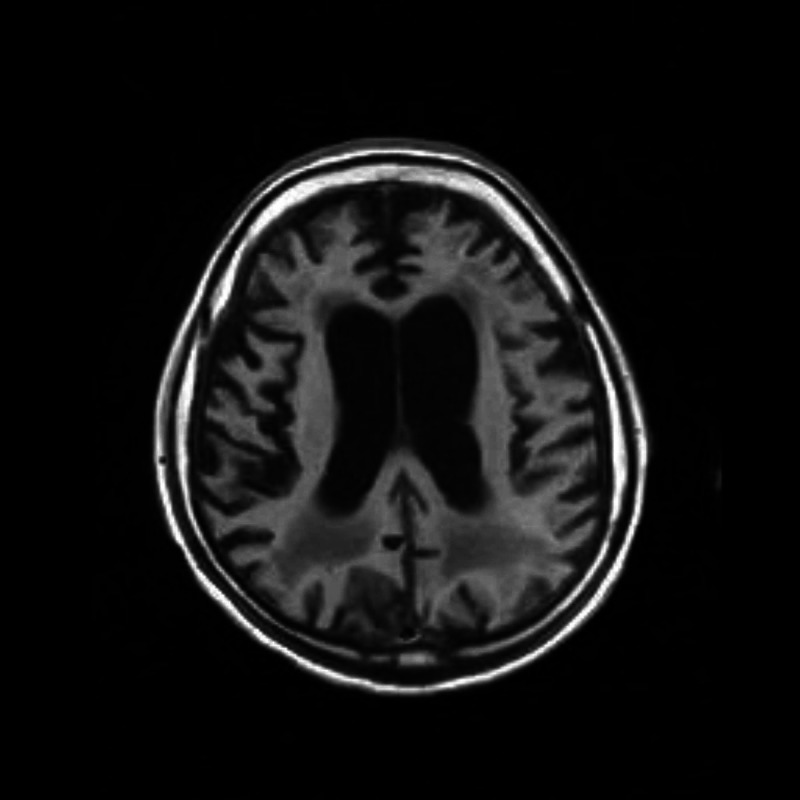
MRI brain showing diffuse cortical atrophy and hydrocephalus MRI: magnetic resonance imaging
